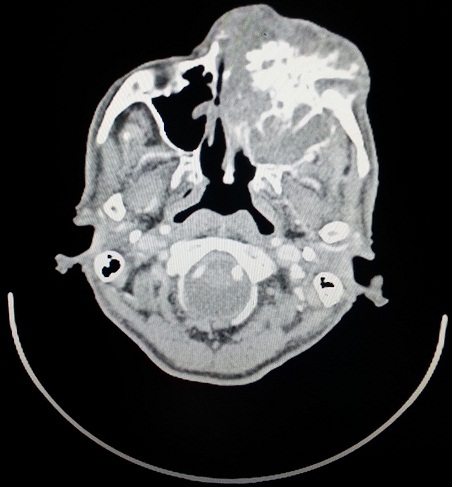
Facial computed tomography showing a large enhancing mass of the left maxillary sinus
